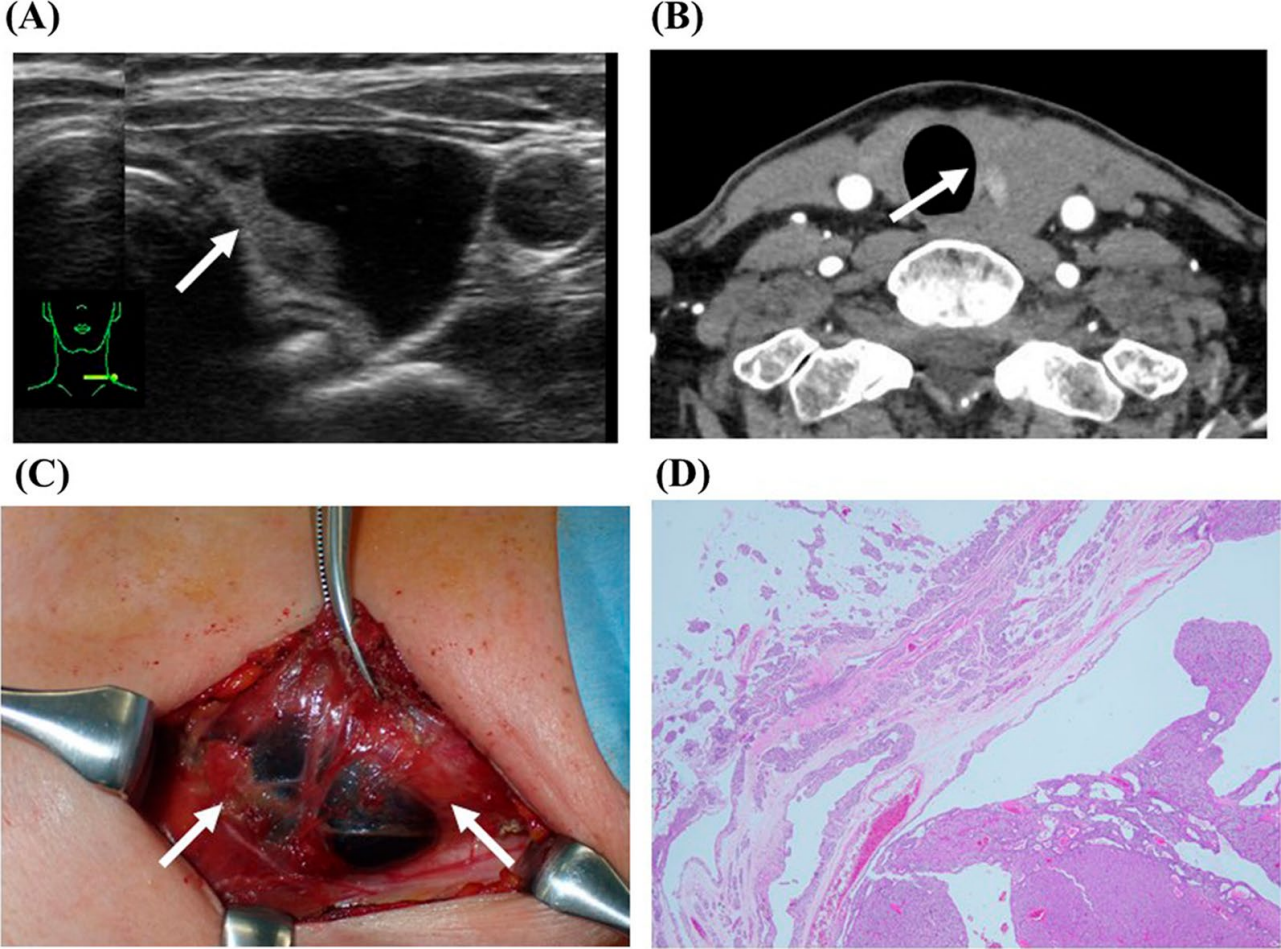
Imaging findings. A Ultrasonography showed a cystic nodular lesion measuring 4.7 × 2.7 × 2.3 cm. It was unclear whether the lesion was located within or outside the left lobe of the thyroid gland. B Contrast-enhanced computed tomography scan showing an enhanced area (white arrow) of the cystic tumor with undeniable findings of cystic parathyroid adenoma. C A 1.4-g parathyroid adenoma is attached to the left lobe of the thyroid gland. D Parathyroid tissue is composed of clear, follicular, cystic, and follicular cells of various sizes and is diagnosed as a parathyroid adenoma
